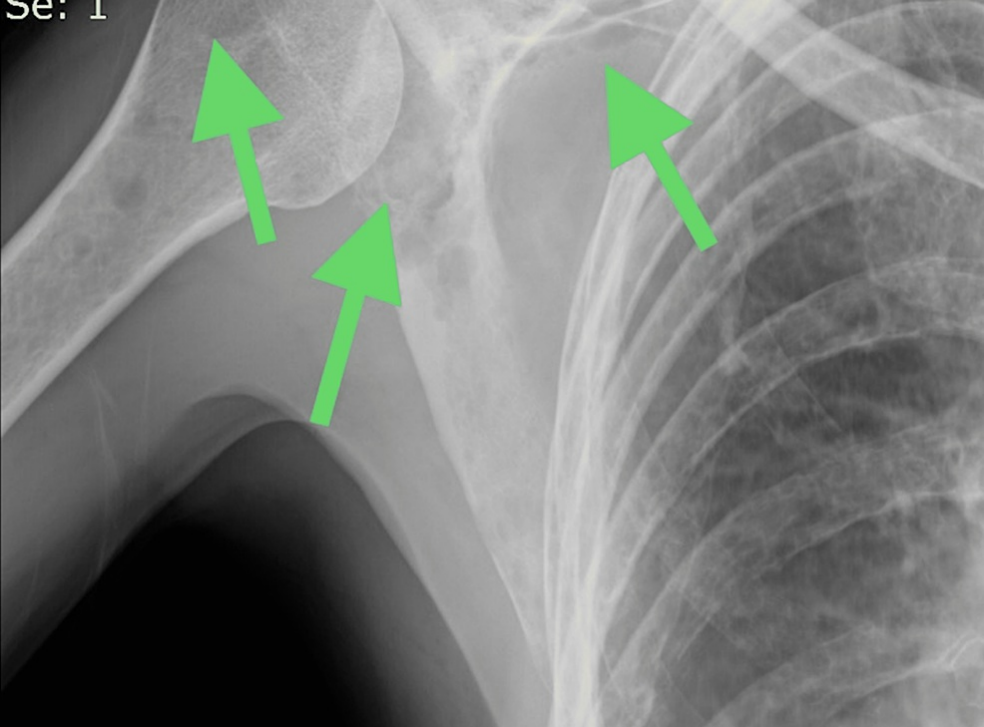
Chest and shoulder X-ray; the arrows indicate lytic lesions in the humerus and scapula.
